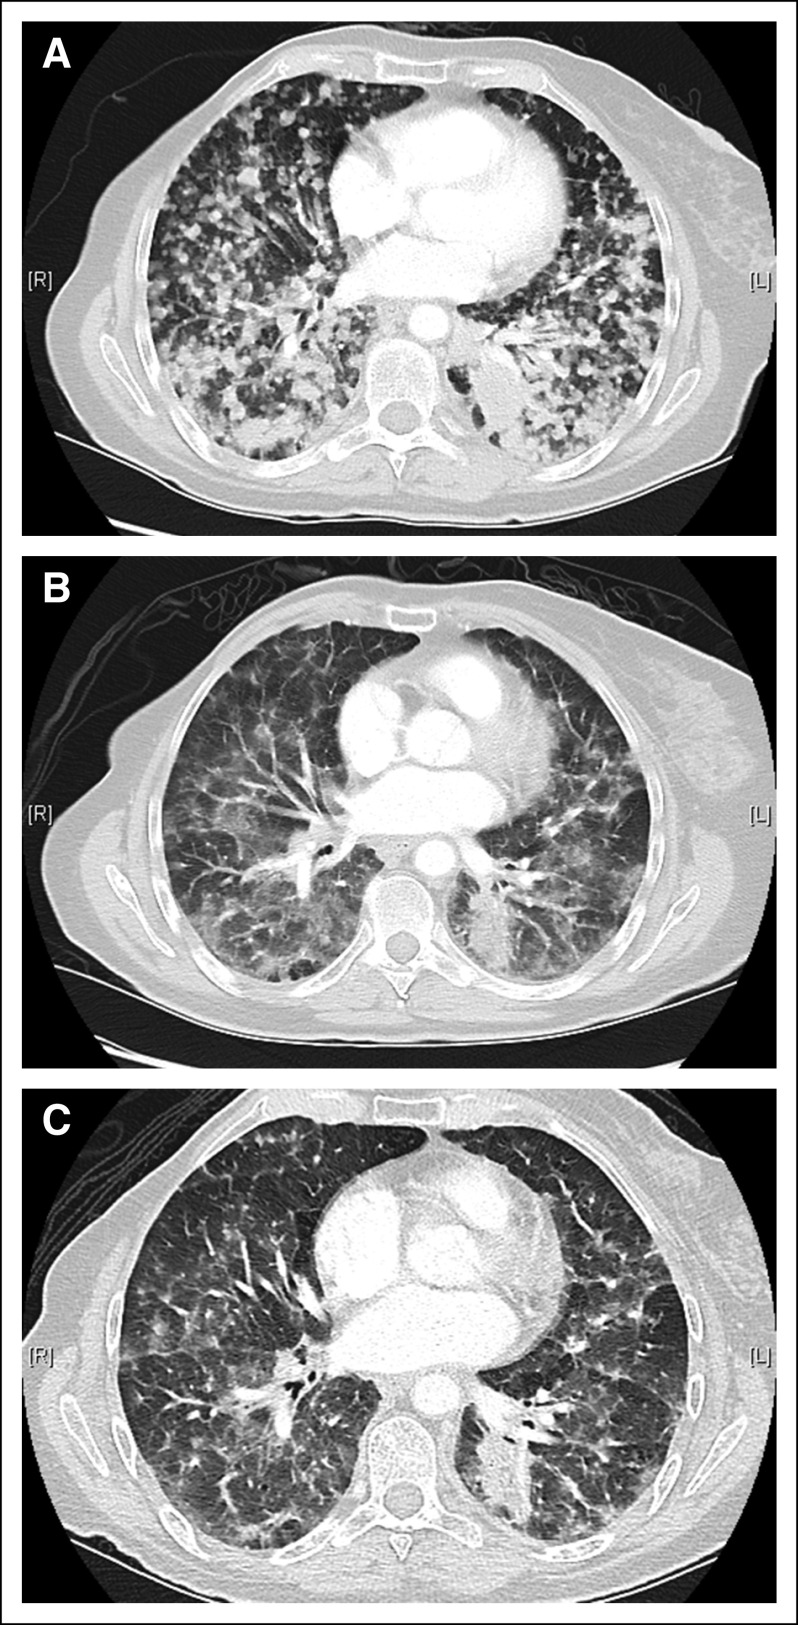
Extracranial response to larotrectinib in a patient with TRK fusion-positive lung cancer. (A) Baseline computed tomography imaging of the chest revealed innumerable bilateral pulmonary metastases that resulted in substantial dyspnea. Treatment with larotrectinib achieved a brisk clinical response within the first 2 weeks as evidenced by decreased shortness of breath. This was accompanied by a radiologic partial response to therapy that (B) was observed at 8 weeks and (C) was confirmed at 16 weeks. Previously solid and semisolid confluent nodules regressed substantially.
